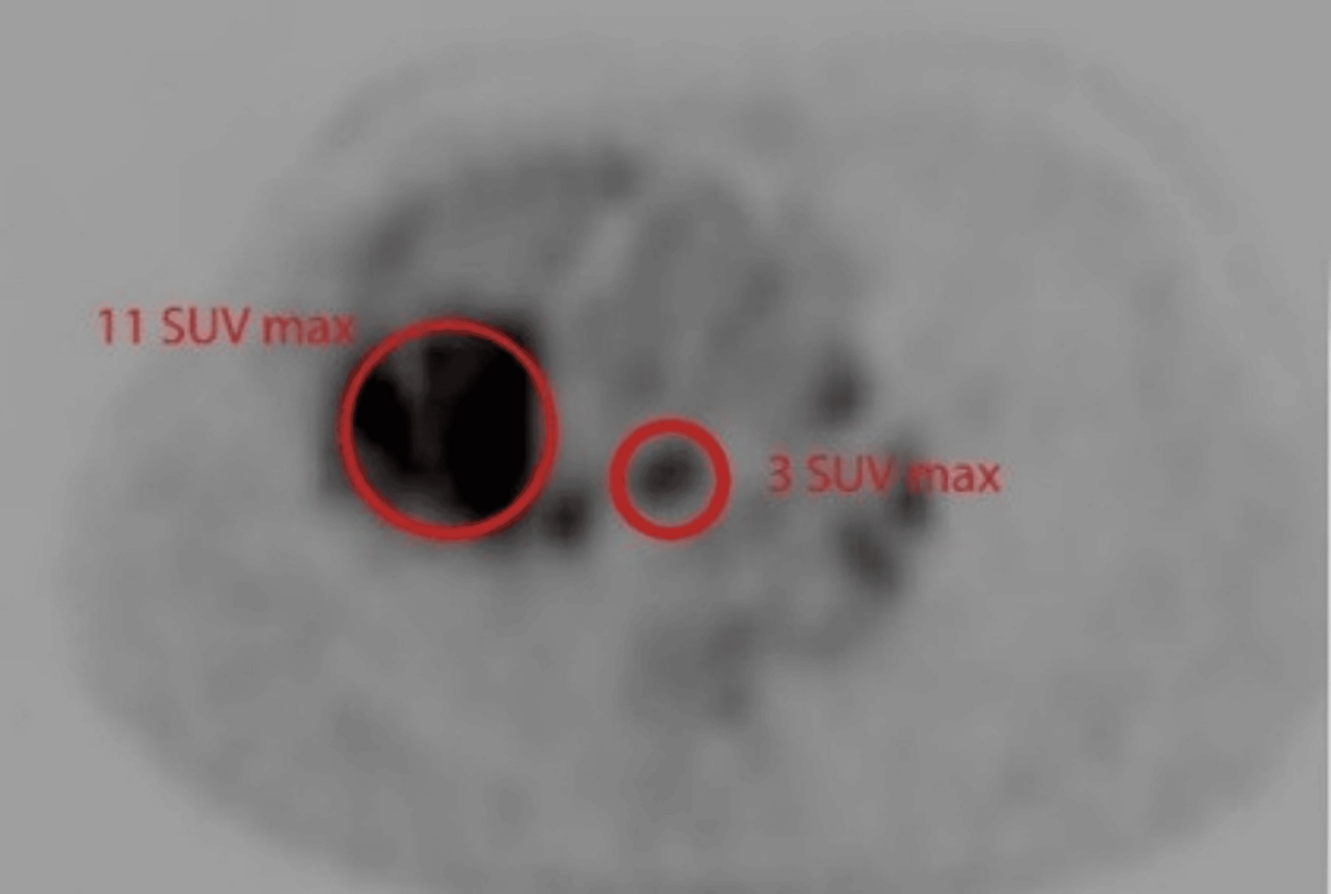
PET component, axial slice, from the same level, showing high metabolic activity in the right perihilar mass as well as in the mediastinal lymph node, with the mass itself showing metabolic activity of 11 SUV, while the maximum SUV of the subcarinal lymph node measures up to 3, indicating a lesser probability of metastasis PET: positron emission tomography; SUV: standardized uptake value
